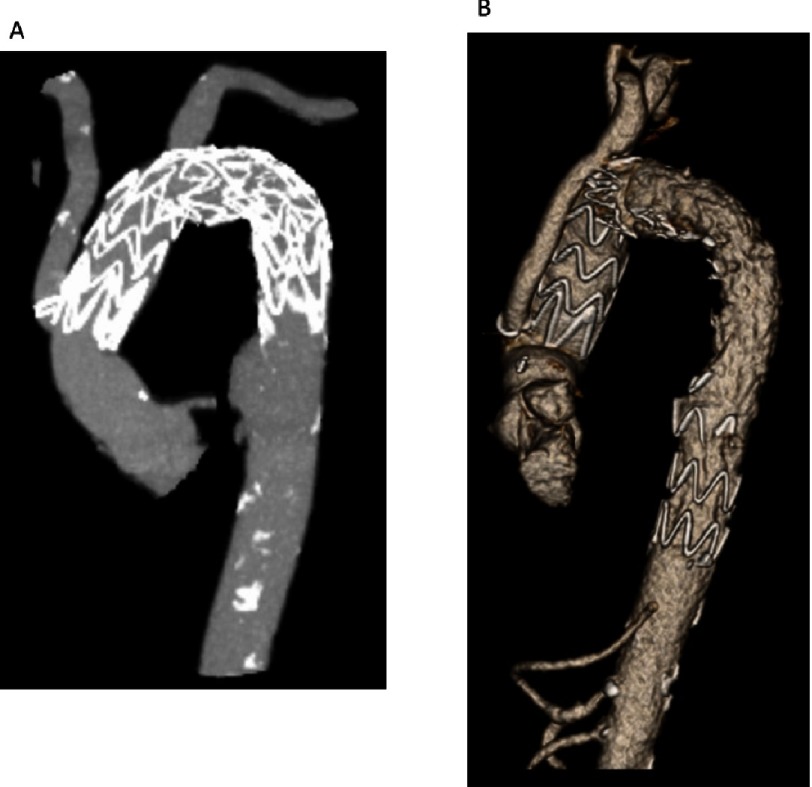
Post-operative CT angiography of the thoracic aorta. (A) Interval CT angiogram re-demonstrated the second saccular aneurysm which had now grown to 2.9 × 3.8 cm, as well as two PAUs. The patient was offered endovascular repair which we performed 13 weeks after the index procedure. (B) He underwent TEVAR extension with 36 × 200 mm TerumoAortic Relay Plus endograft with use of a spinal drain. Completion angiogram revealed no endoleak and the final CT shows exclusion of the second aneurysm and PAUs.
